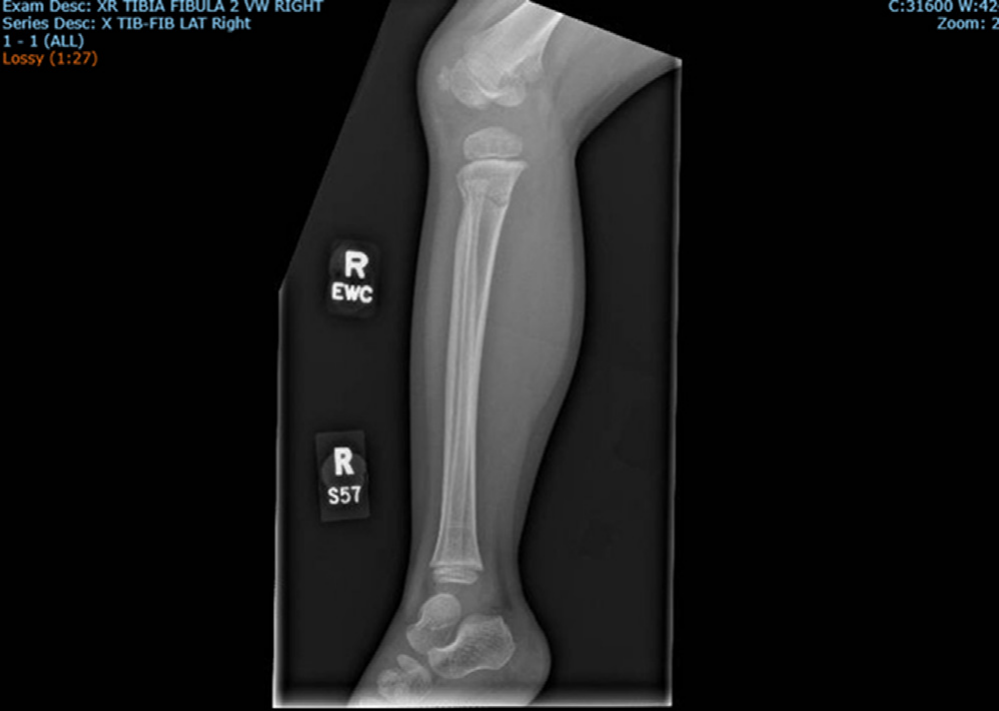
Lateral plain film of right lower limb.
